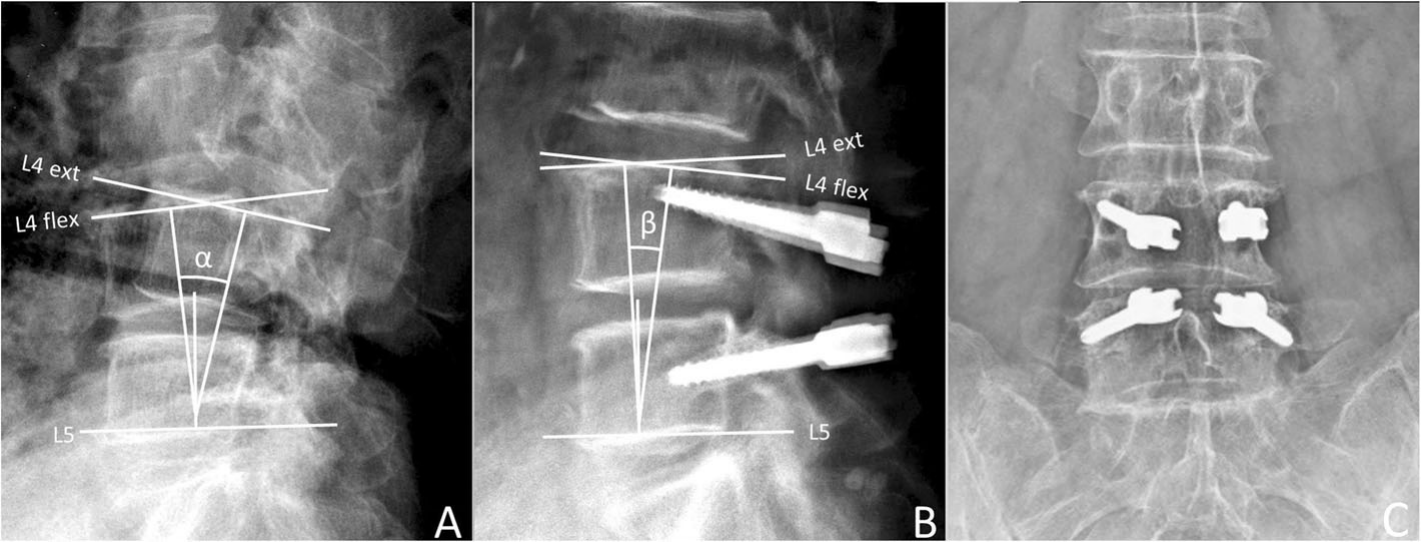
Comparison of pre-operative (a) and post-operative (b) dynamic (flexion/extension) lateral radiographs. Pre-operative ROM α:15.41°, Post-operative ROM β:3.1°. c Post-operative anterior-posterior fluoroscopy
